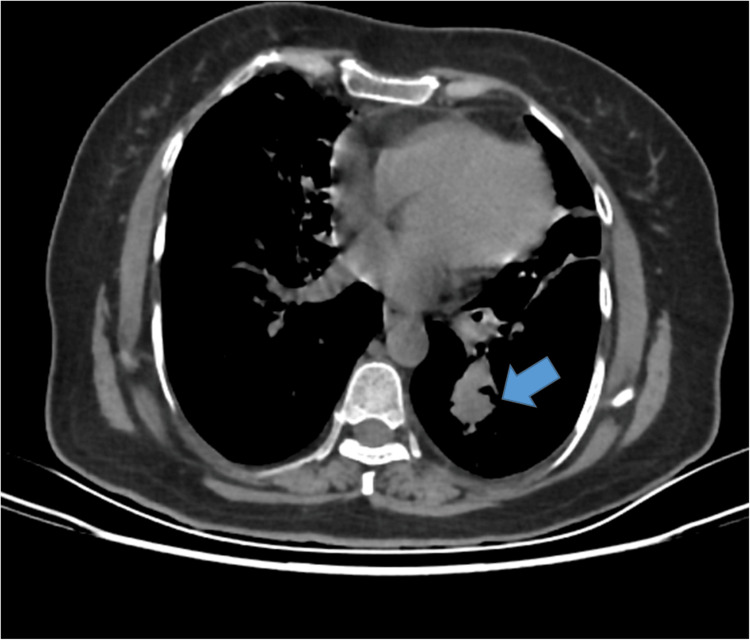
CECT of the thorax showing ill-defined soft tissue density in the left lung suggestive of pulmonary metastasis CECT: contrast-enhanced computed tomography
